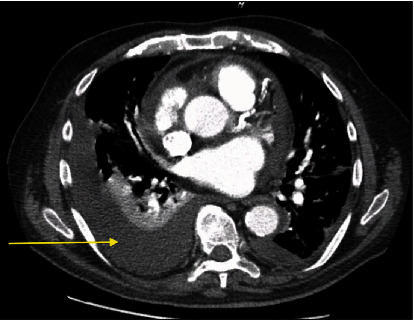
CT scan showing left-sided pleural effusion (yellow arrow).
